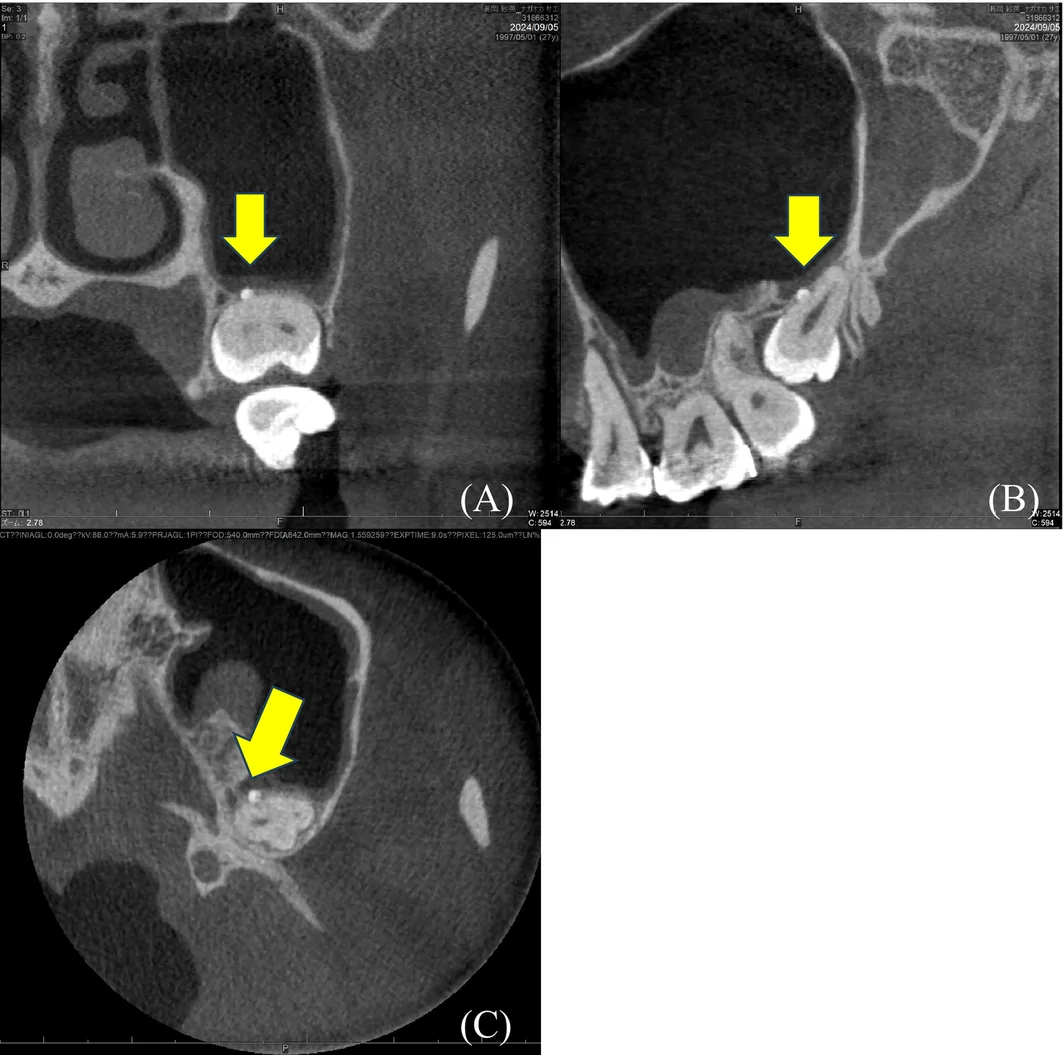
Cone‐beam computed tomography images. Image acquired on September 5, 2024, using 3D Accuitimo (J. MORITA Corp, Osaka, Japan) under the usual imaging conditions used at our clinic (a tube voltage of 80 kV, a tube current of 5.0 mA, an FOV of Φ60 × H60 mm). (A) Coronal slice image, (B) Sagittal slice image, (C) Horizontal slice image. A semicircular, highly absorbent image of an enamel pearl was observed in the area indicated by the arrow.
